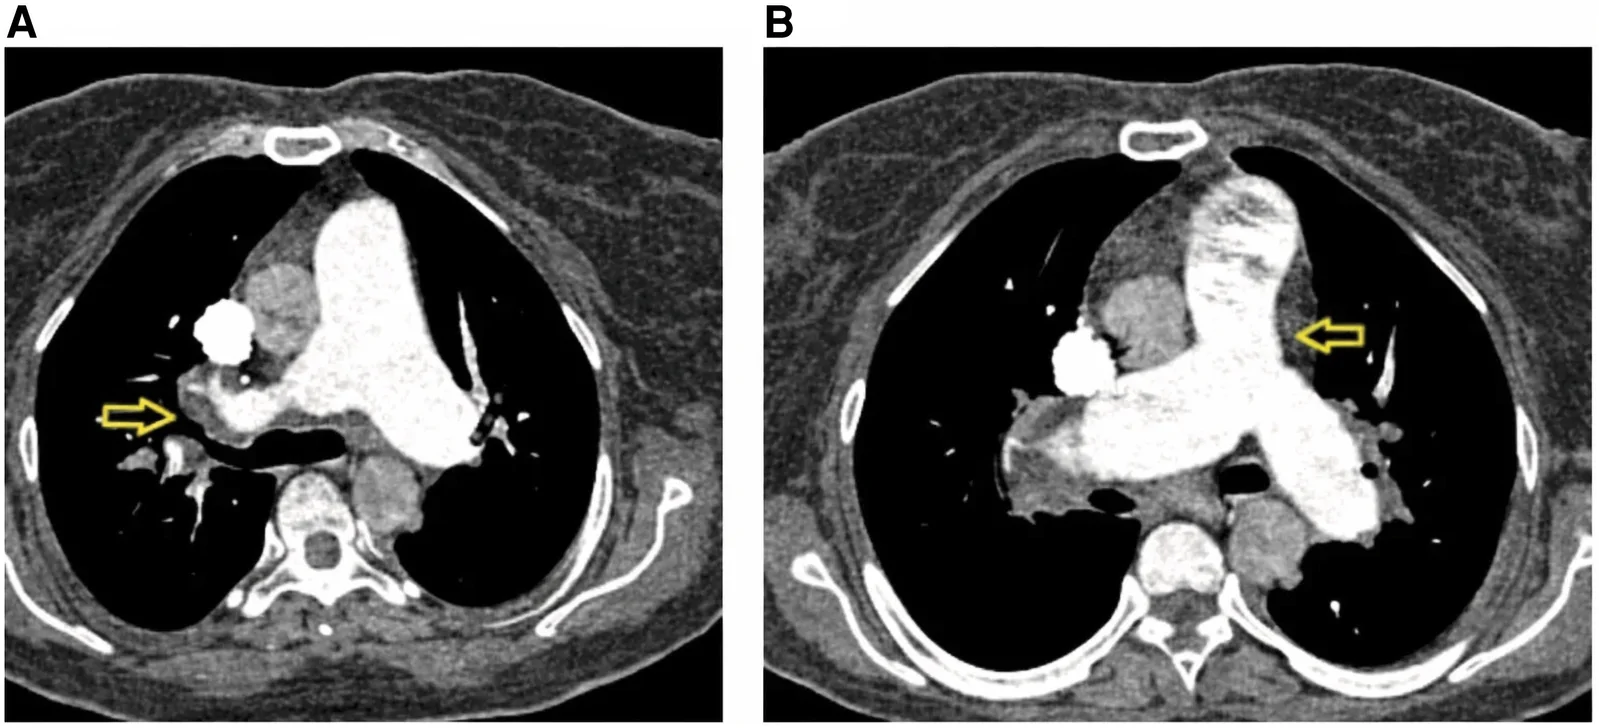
Contrast-enhanced computed tomography pulmonary angiography (CTPA) demonstrating pulmonary embolism. Intraluminal filling defects are visible within segmental branches of the pulmonary arteries (arrows). Panels (*A*) and (*B*) show embolic material in different pulmonary arterial branches, consistent with pulmonary embolism.
